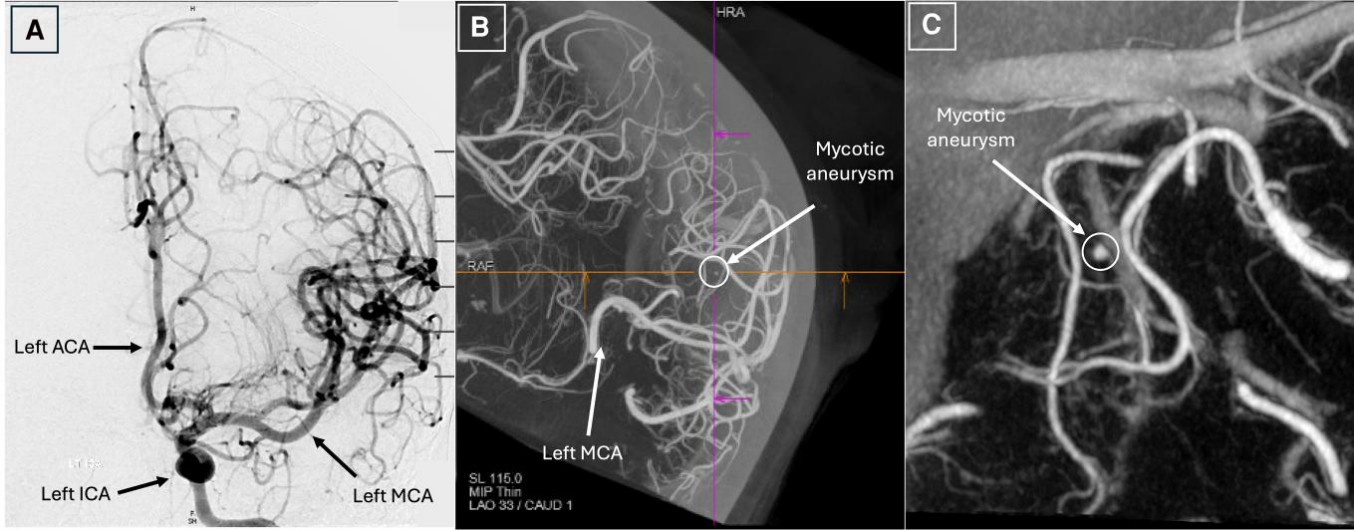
Case 1 (Mycotic Aneurysm): cerebral angiography in coronal plane with Dyna-CT reconstruction sequences. During cerebral angiography, contrast is injected into the internal carotid artery (*Panel A*). Due to the exceedingly small size of mycotic aneurysms, aneurysms are difficult to identify on digital subtraction angiography. However, through Dyna-CT, a technology used to create computed tomography-like images in the angiography suite, smaller vascular abnormalities, like mycotic aneurysms, can be better visualized. The round, protruding opacity seen on DynaCT (*Panels B & C*) represents an aneurysm in the M4 segment of the inferior division of the left middle cerebral artery. In the context of positive blood cultures and infective endocarditis, this is concerning for a mycotic aneurysm. ACA, anterior cerebral artery; ICA, internal carotid artery; MCA, middle cerebral artery.
